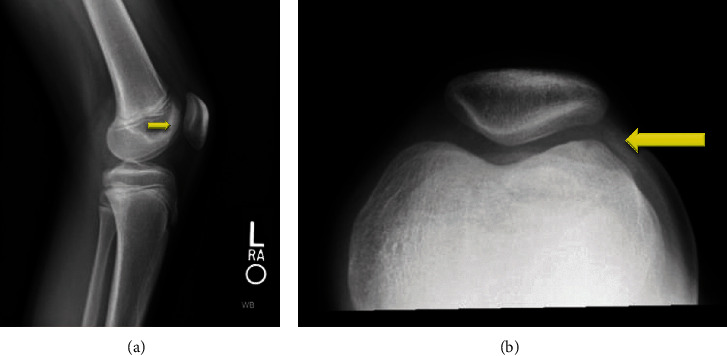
Initial X-rays of the patient showing osteochondritis dissecans (OCD) lesion of the lateral trochlear groove on (a) lateral and (b) sunrise views of the left knee.
